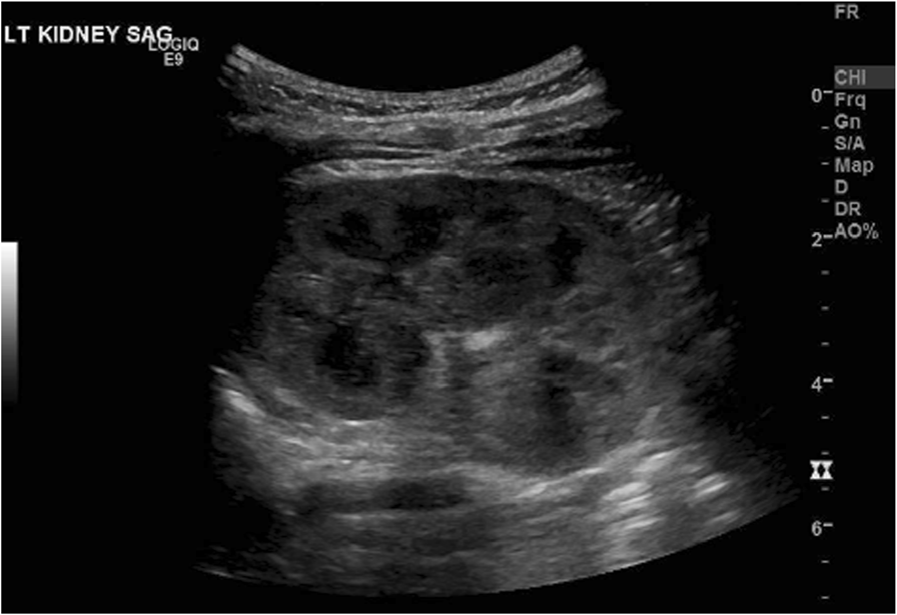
Renal Ultrasound of Right Kidney-5.1 × 2.5 × 2.9 cm
